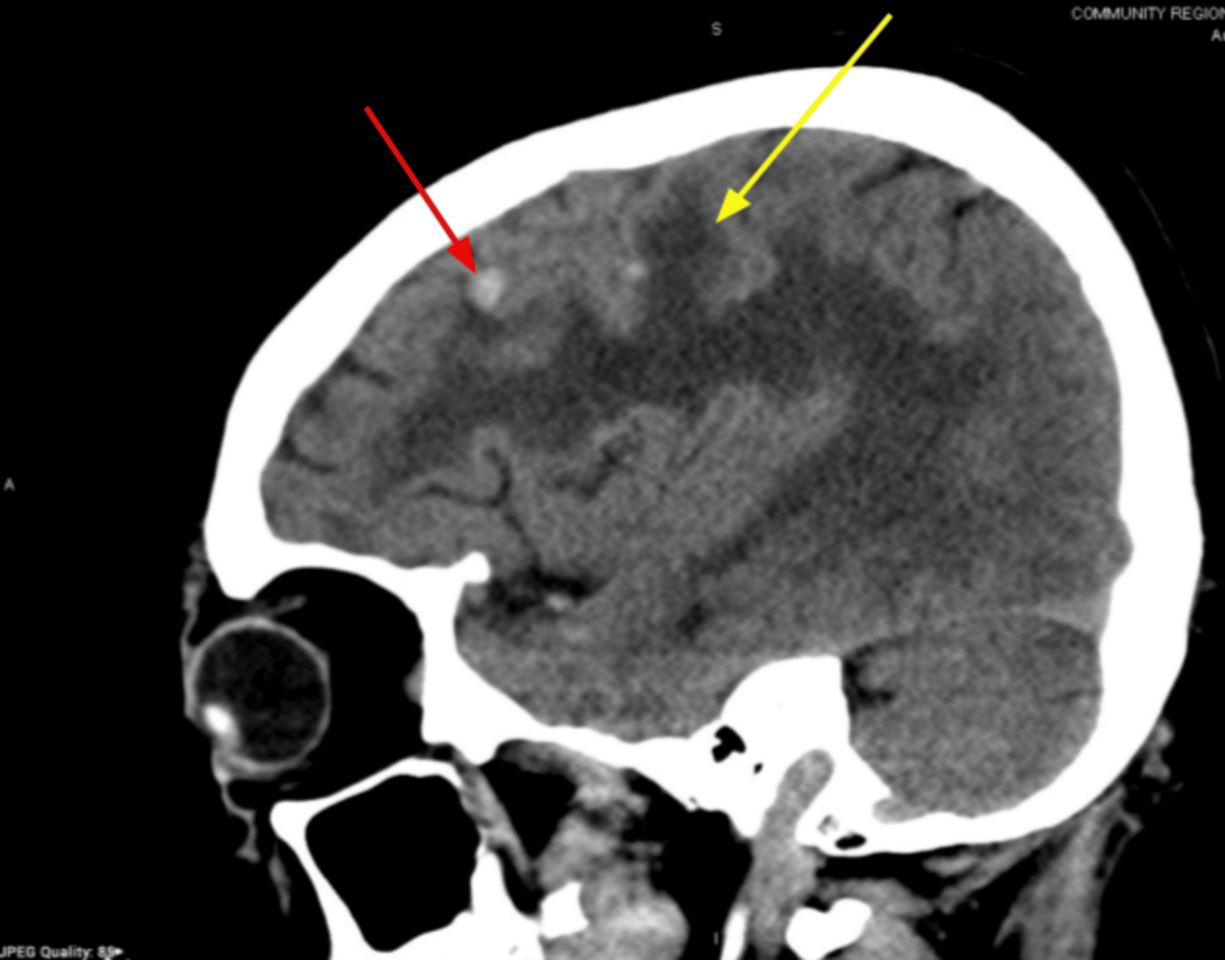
Brain MRI (October 2023) showing a left parasagittal frontal lobe lesion (red arrow) and surrounding vasogenic edema (yellow arrow)
